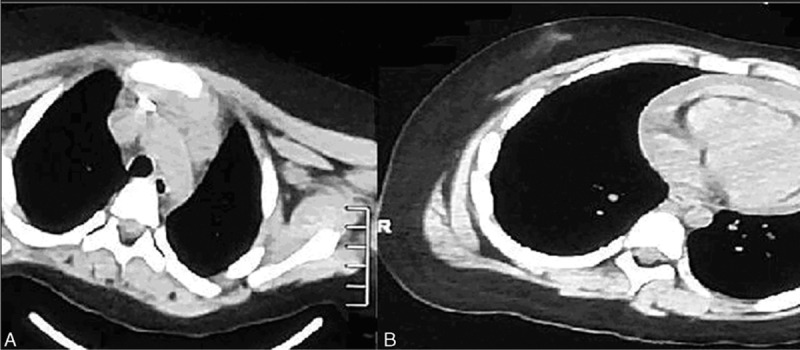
(A) CT scan showing that the migrated Kirschner wire was near the wall of ascending aorta. (B) CT scan showing massive hemopericardium and pericardial tamponade. CT scan = computed tomographic scan.
